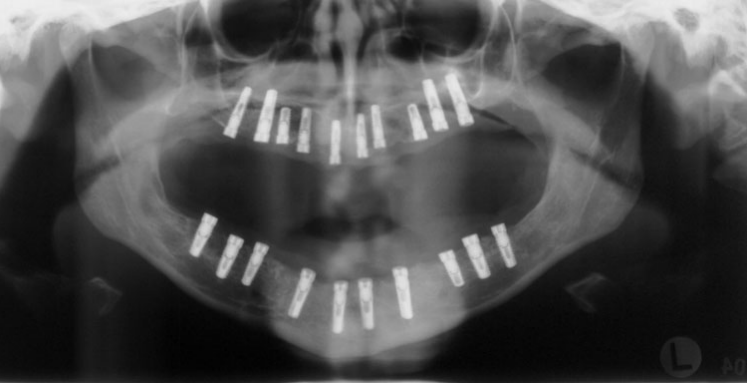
postoperative panoramic radiographs after implant setting and bone augmentation.
